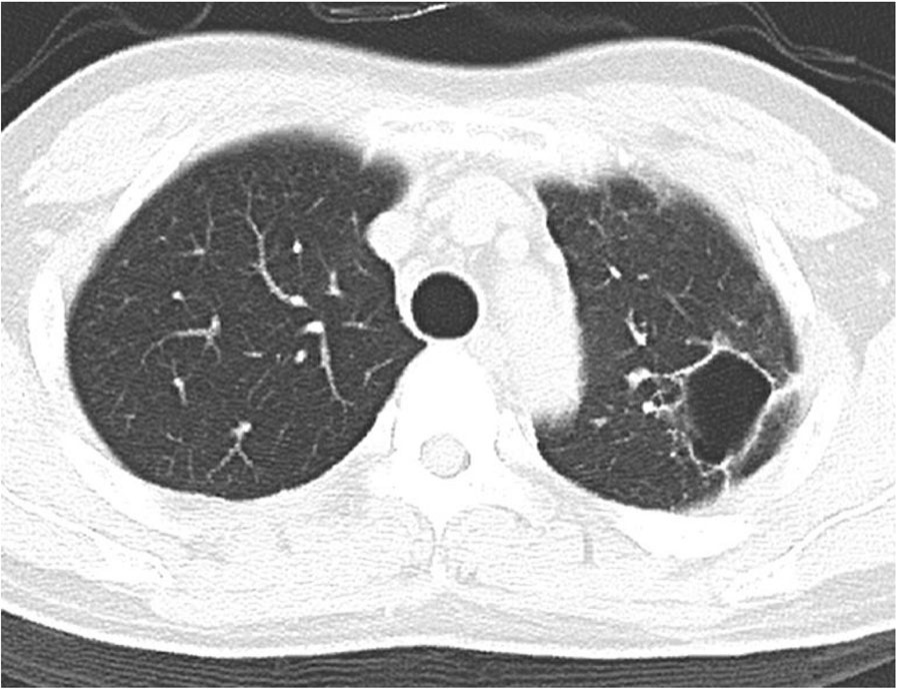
Computed tomography findings after 7 months. The lung abscess is completely cured with a small residual thin wall cavity
